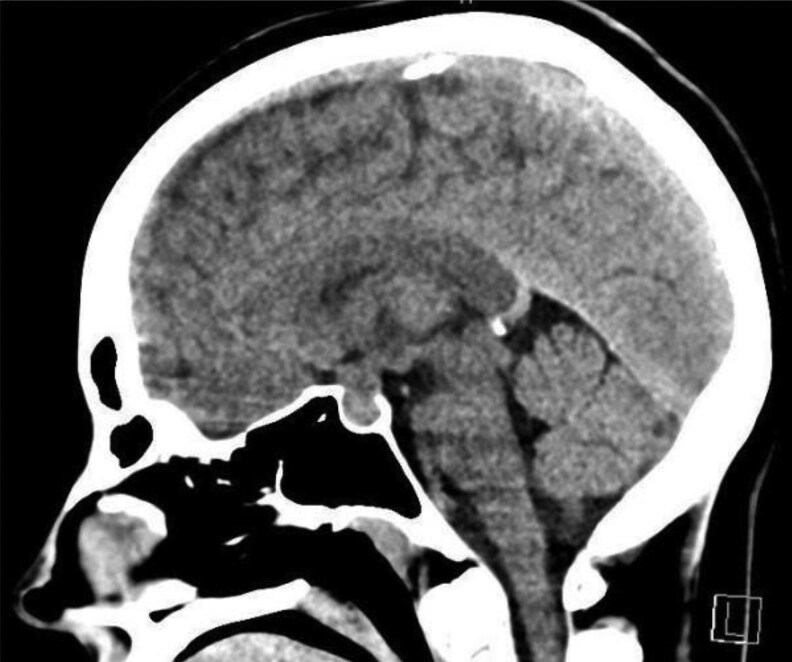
Figure shows a noncontrast computerized tomography (CT) in the sagittal plane of the brain at time of presentation with vomiting, photophobia, and headache in October 2023.
